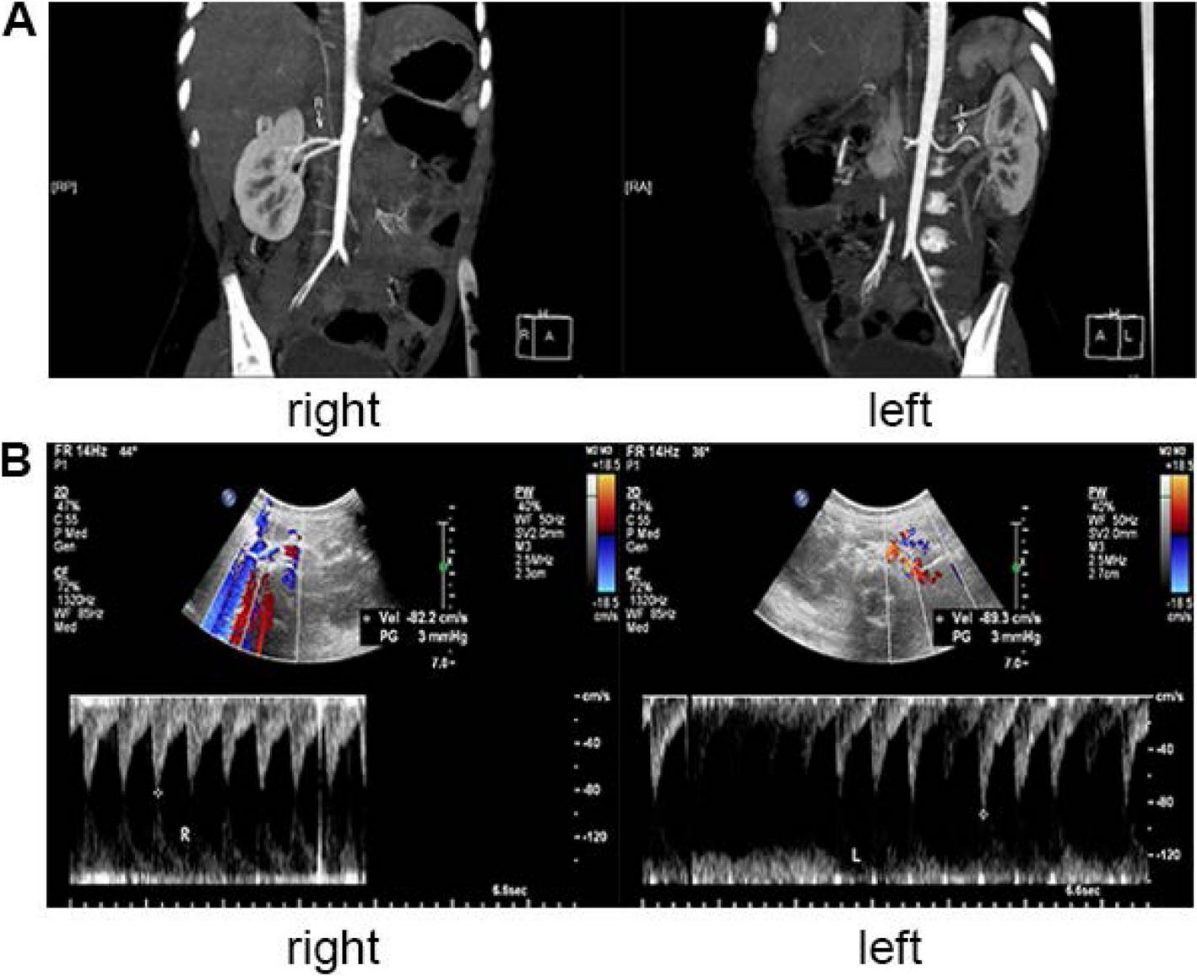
Contrast-enhanced computed tomography of the abdomen and renal artery Doppler ultrasound. a contrast-enhanced computed tomography of one-and-a-half-year-old girl: the abdomen image shows normal renal arterial (white arrow); b Renal artery Doppler ultrasound: the internal diameter for the right renal artery was 2.2 mm at the beginning, the maximum forward blood flow velocity was 89.3 cm/s (in normal range: 69.61 ± 17.77 cm/s); the internal diameter for the left renal artery was 2.5 mm at the beginning, the maximum forward blood flow velocity was 82.2 cm/s (in normal range: 69.61 ± 17.77 cm/s)
